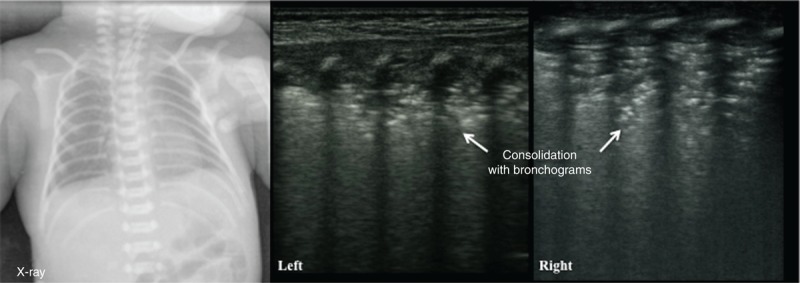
Ultrasonic manifestation of RDS: pulmonary consolidation with air bronchograms. The infant above had a GA of 39^+1^ wk and was delivered by cesarean section; the infant had a birth weight of 4000 g. The infant was admitted to the hospital because of dyspnea for 4 h. The CXR indicated RDS. LUS showed large-area lung consolidation with significant air bronchograms in bilateral lungs and the disappearance of the pleural lines and A-lines. CXR = chest radiography, GA = gestational age, LUS = lung ultrasonography, RDS = respiratory distress syndrome.
